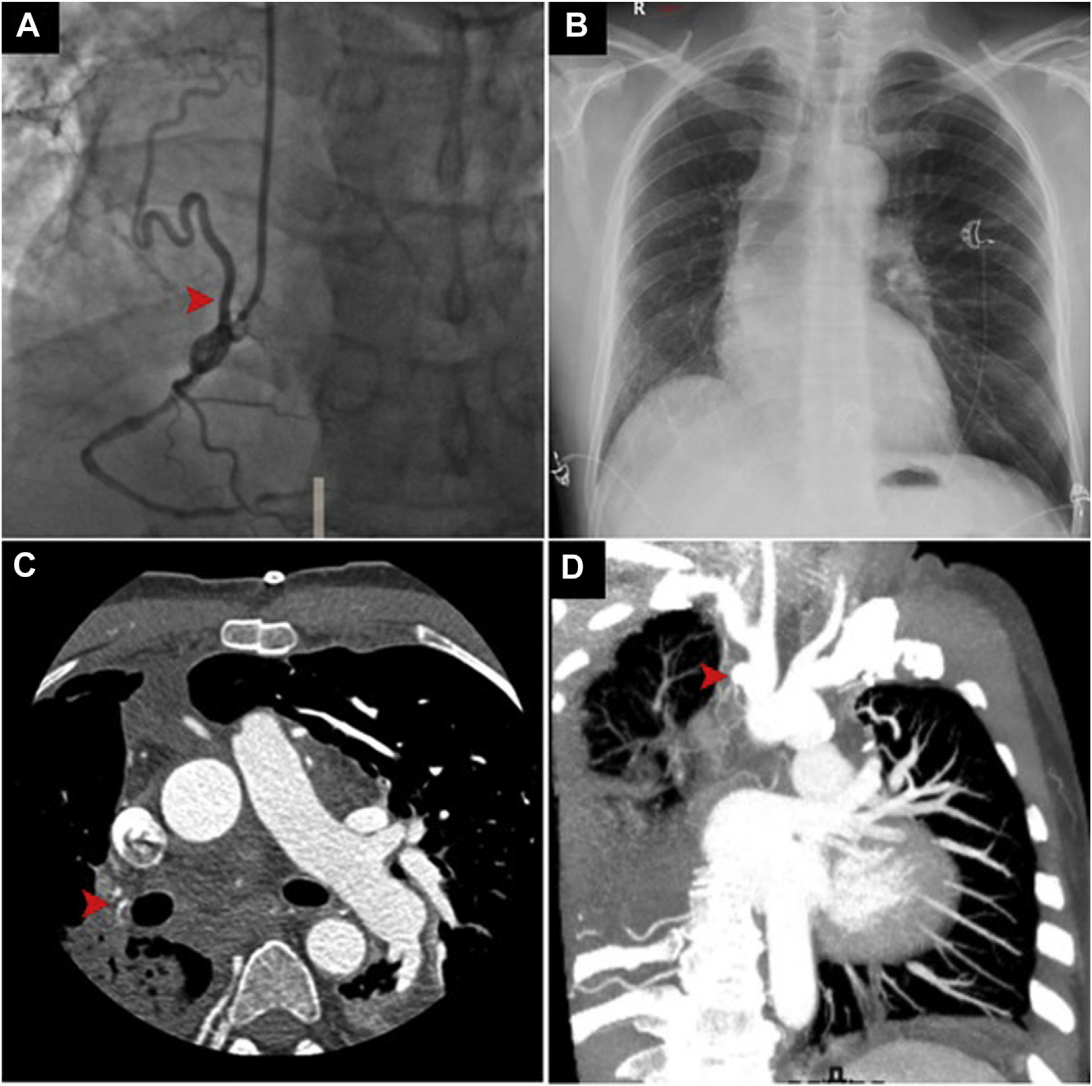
(A) Right coronary angiogram revealing an anomalous collateral originating from the right coronary artery (arrowhead). (B) Chest radiograph showing asymmetric lung volumes, mediastinal shift towards the right and ipsilateral elevated hemidiaphragm. (C) Axial angiogram demonstrating proximal interruption of the right pulmonary artery while the distal pulmonary arterial tree is maintained (arrowhead). (D) Coronal angiogram showing the telltale sign of ductal origin of pulmonary artery, namely, the ductal diverticulum (arrowhead).
